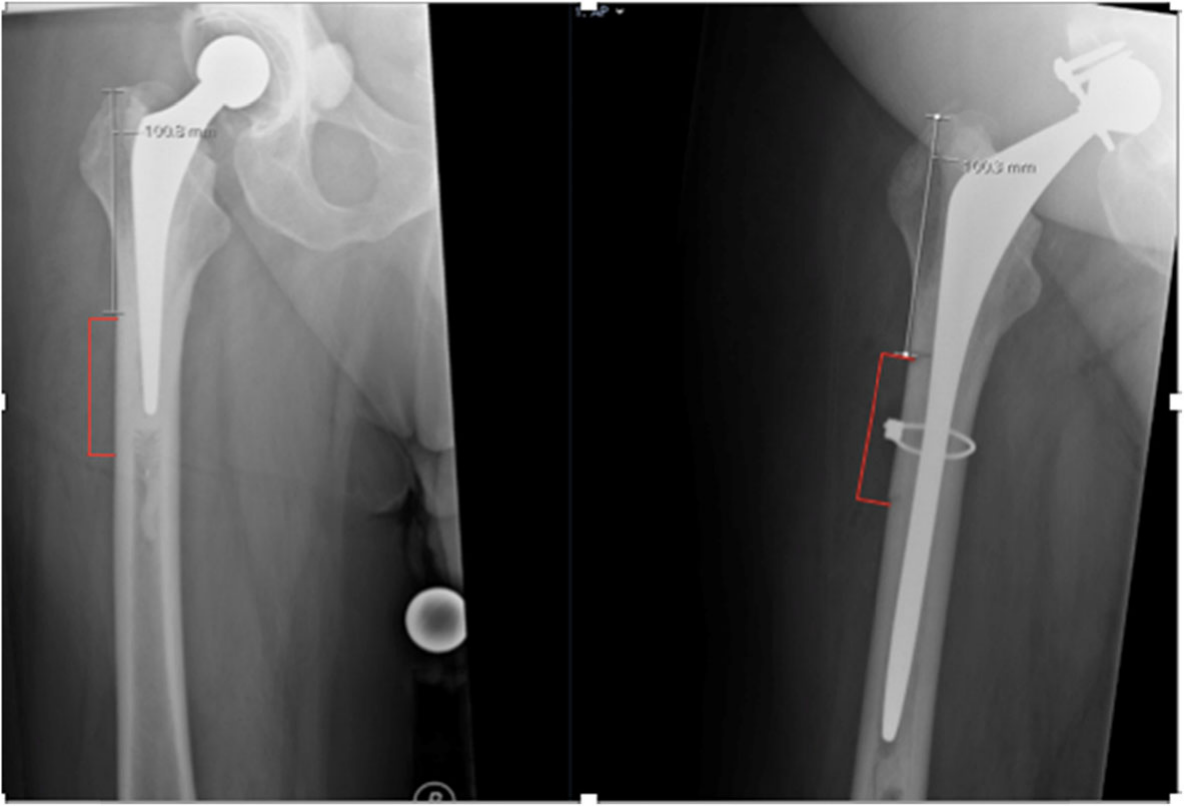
Radiographs of a patient with infected cemented THR, marked preoperatively for osteotomy site. Note the starting point of osteotomy site measured from the tip of the greater trochanter
